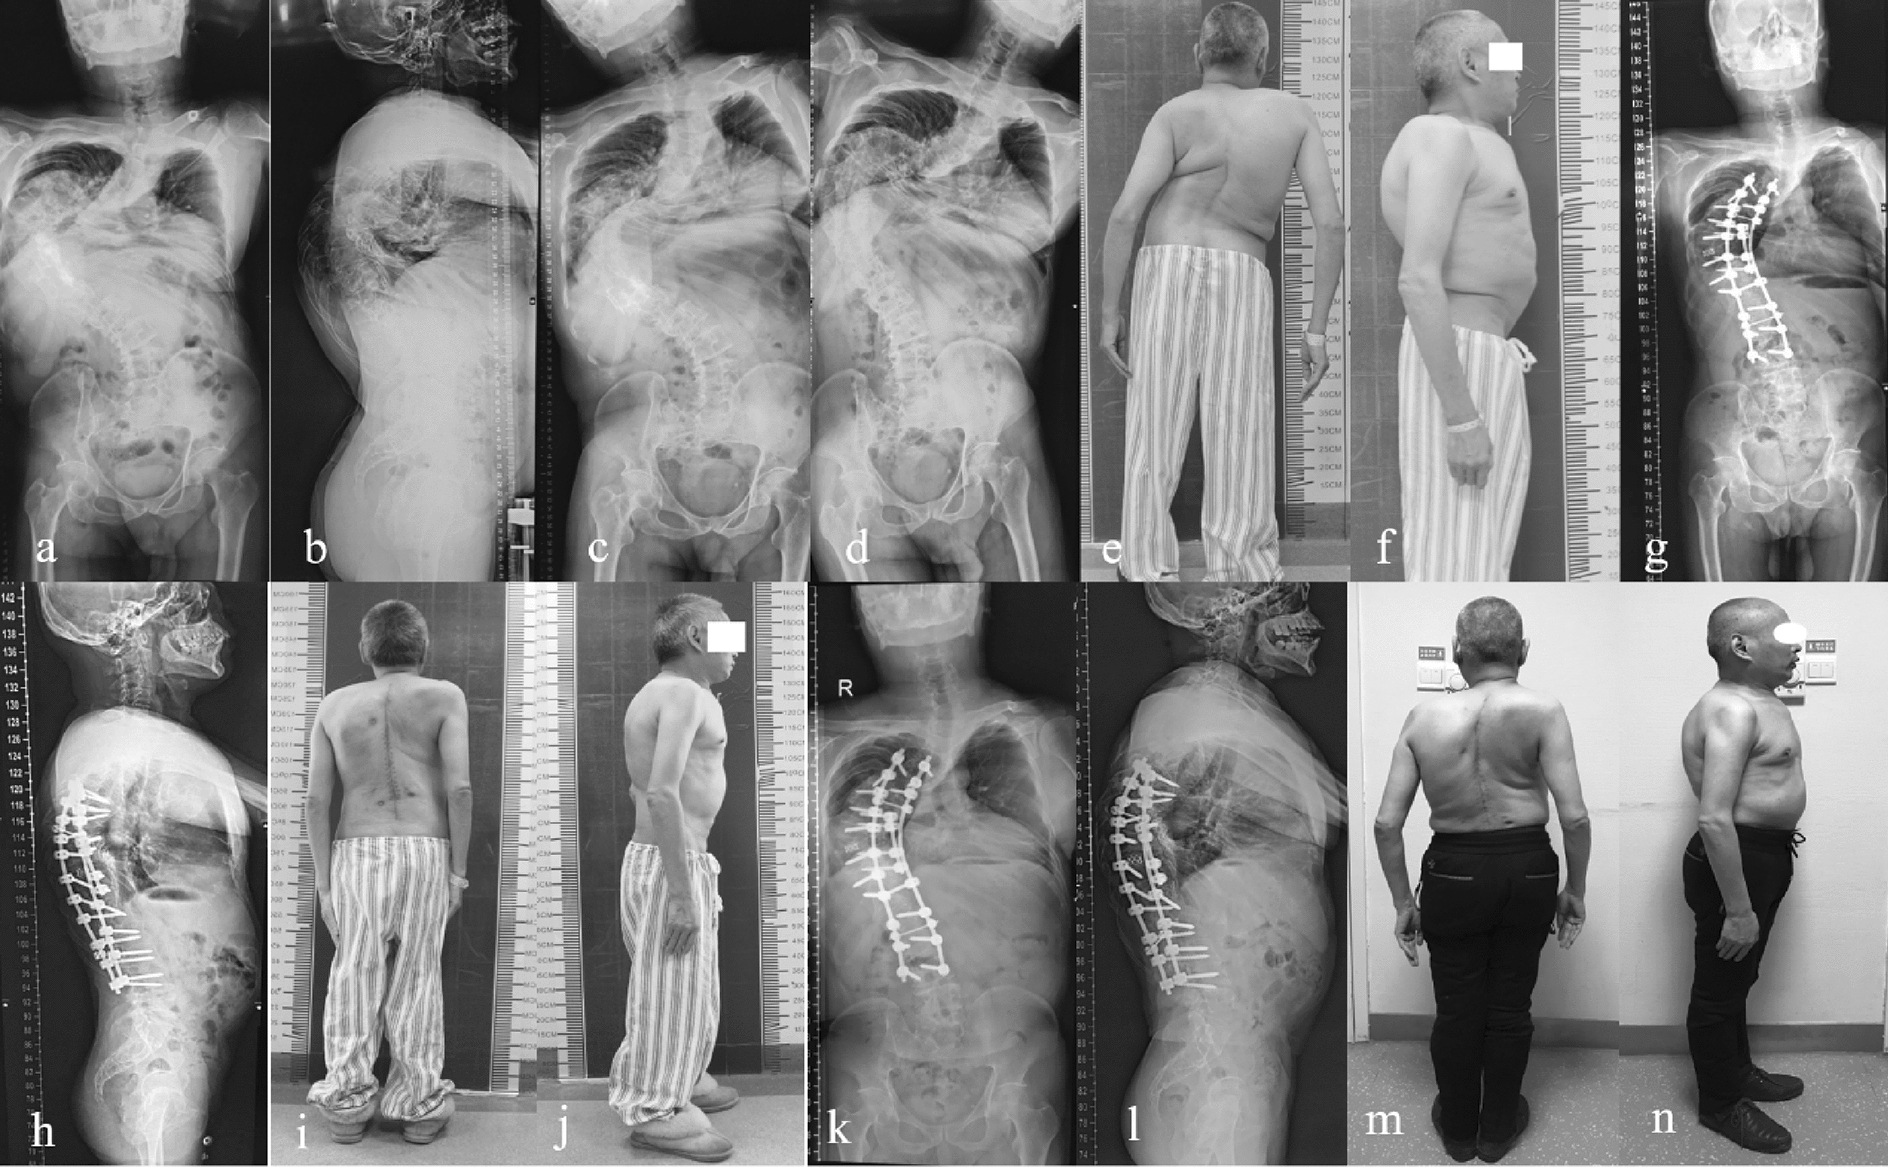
Standing radiographs and images of a 57-year-old female with severe rigid spinal deformity. a–d Preoperative radiographs; e–f preoperative appearance in posterior and lateral view; g–h postoperative anterior–posterior and lateral radiographs; i–j postoperative appearance in posterior and lateral view; k–l anterior–posterior and lateral radiographs at the final follow-up; m–n posterior and lateral appearance at the final follow-up
